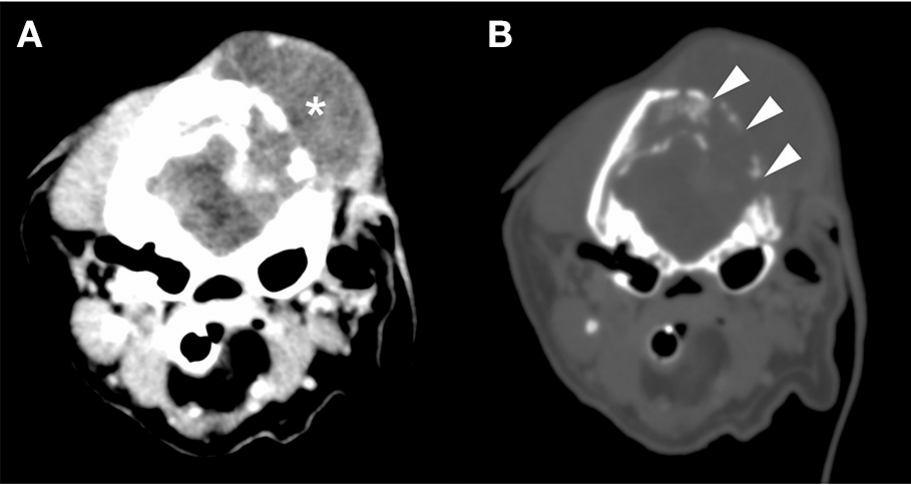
Cranial CT images [(A) bone conditions, (B) soft tissue conditions]. Contrast-enhanced CT image of the head. Soft tissue mass formation was observed mainly in the left temporal-occipital region (*). Furthermore, the region showed destruction of the occipital bone (arrowhead) and an unclear boundary with the brain parenchyma. No obvious metastatic findings were found in the chest or abdomen. CT, computed tomography.
